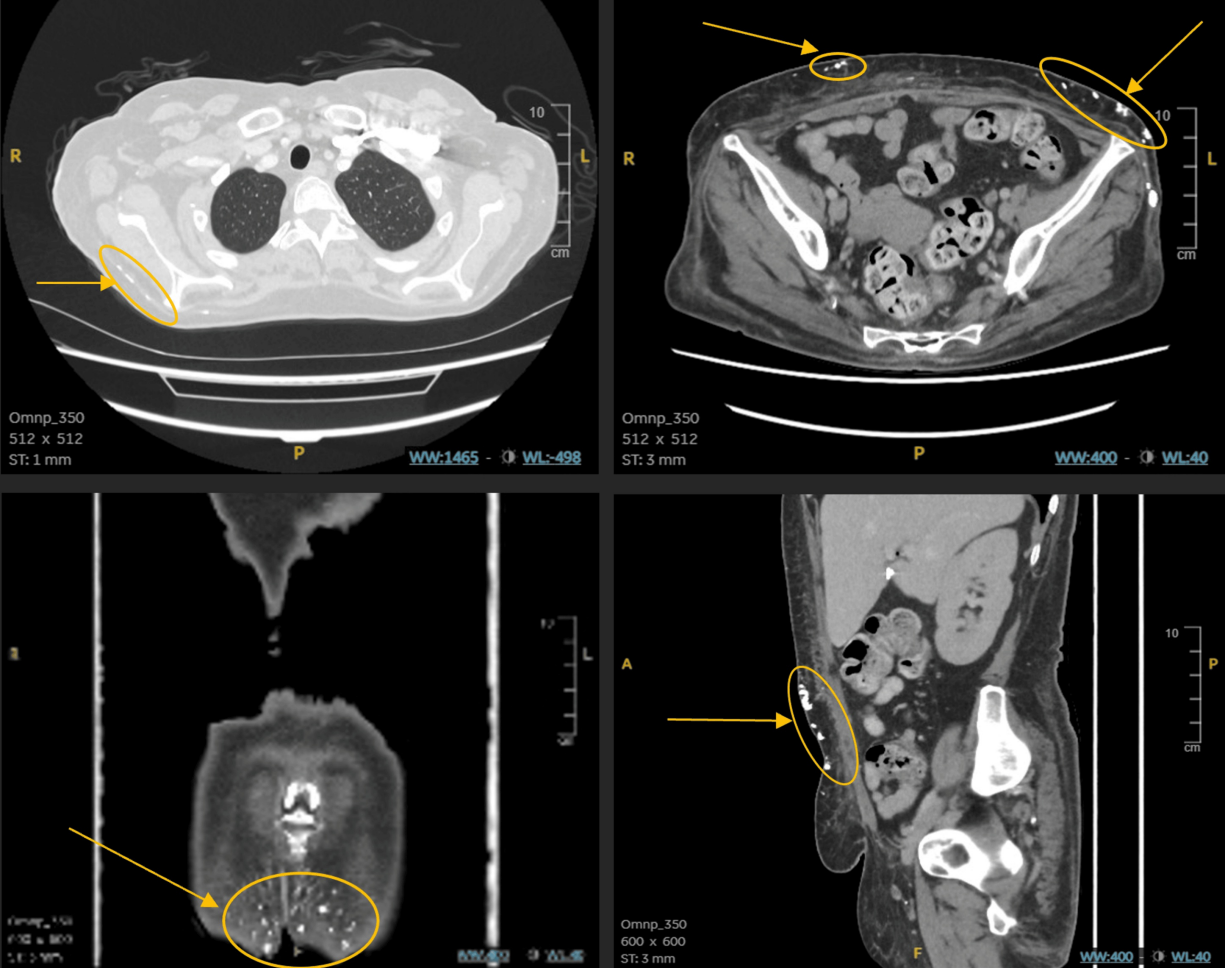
The neck-chest-abdomen-pelvis CT showing extensive calcinosis cutis affecting the chest, abdomen, and pelvic region.
